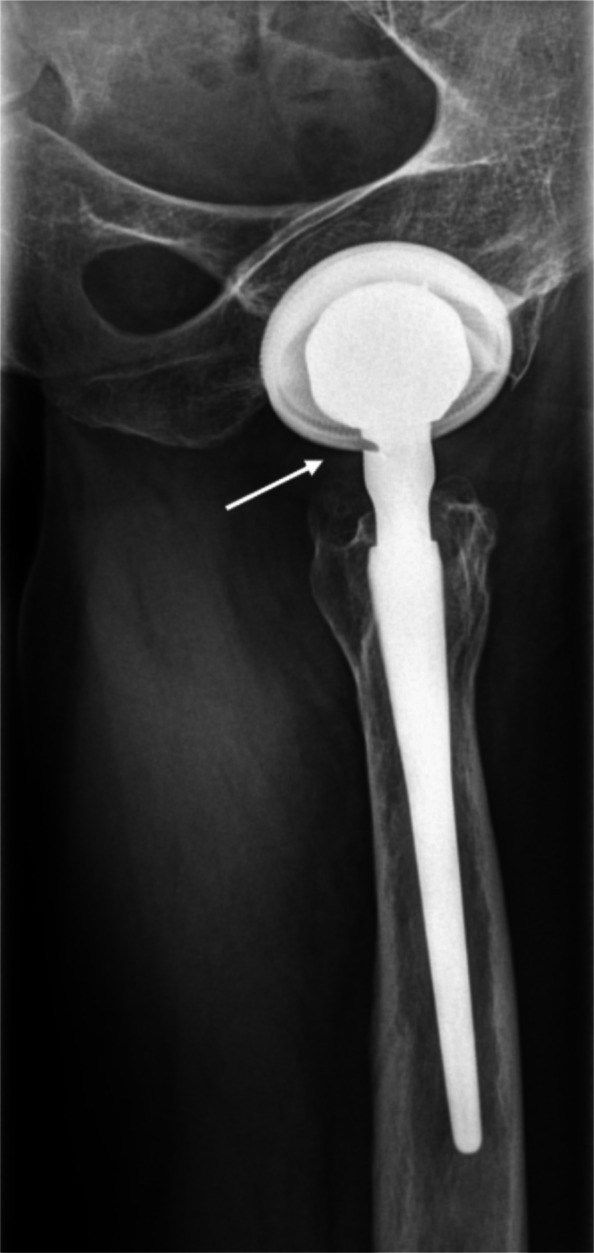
Axial radiograph of the left THA of a 60-year-old female patient, taken 25 years post index-surgery, demonstrating severe notching of the implant stem
